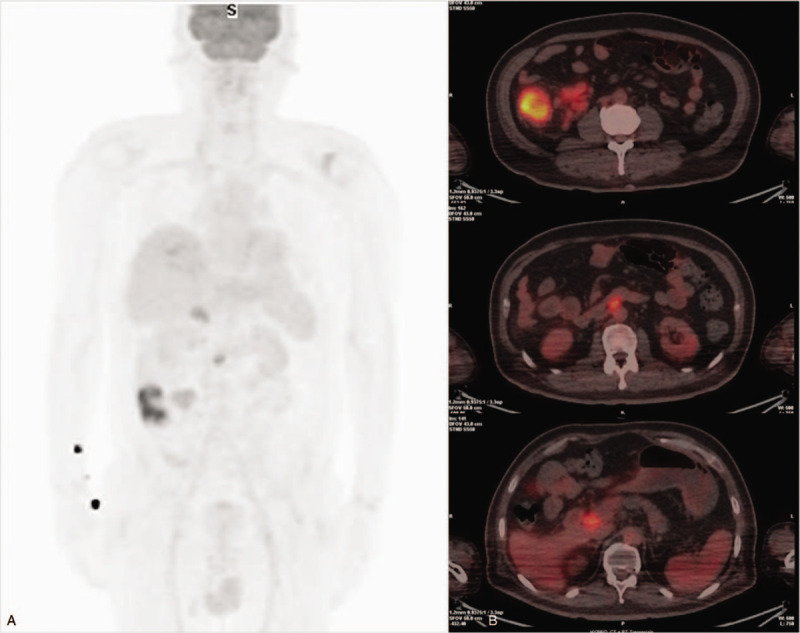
(A and B) ^18^F-fluorodeoxyglucose (FDG) positron emission tomography/computed tomography at presentation shows wall thickening with increased ^18^F-FDG uptake (maximum standardized uptake value 13.55) in ascending colon and enlarged lymph nodes with increased ^18^F-FDG uptake (7.09) in pericolic, aortocaval, and porta hepatis areas. FDG = fluorodeoxyglucose.
